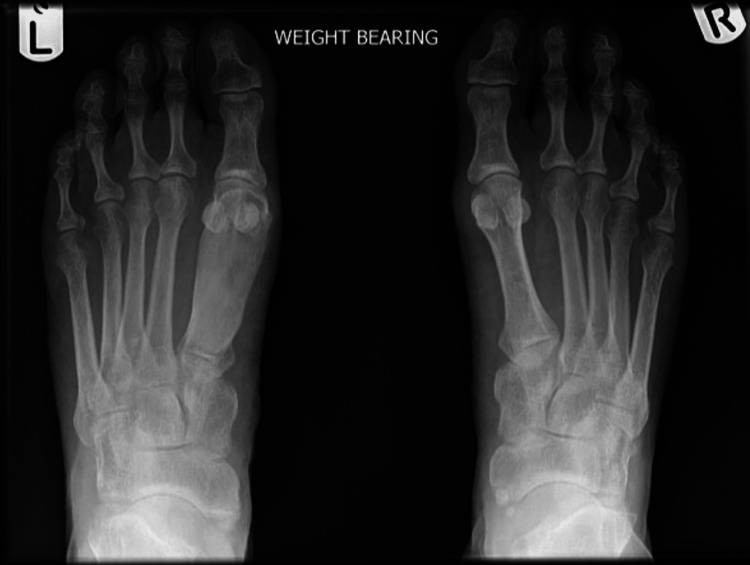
Anteroposterior pre-operative X-ray showing arthritic changes in the left metatarsophalangeal (MTP) joint.
